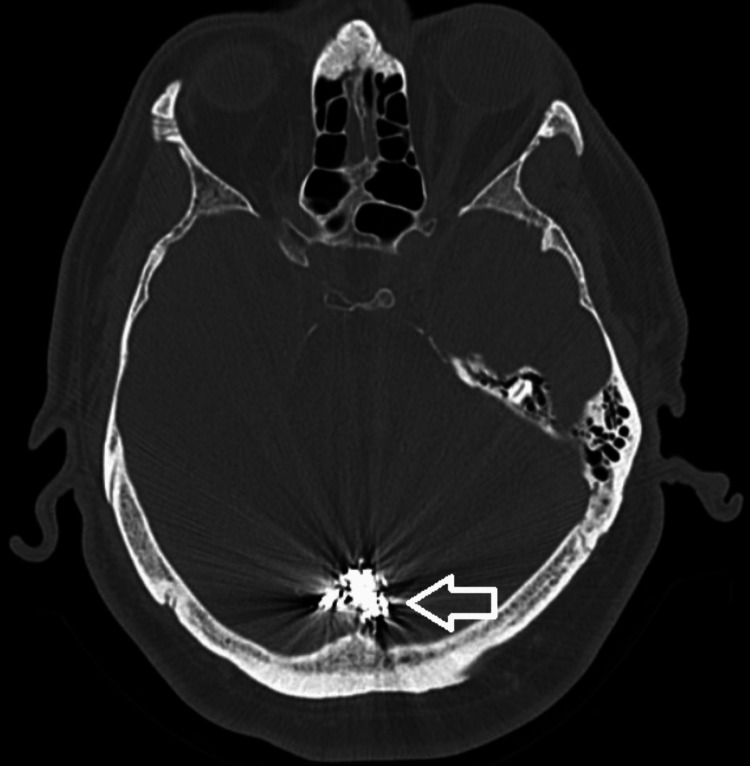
An axial cut of the CT head (bone window), with an arrow pointing to the dense Onyx cast in the posterior fossa. CT, computed tomography
